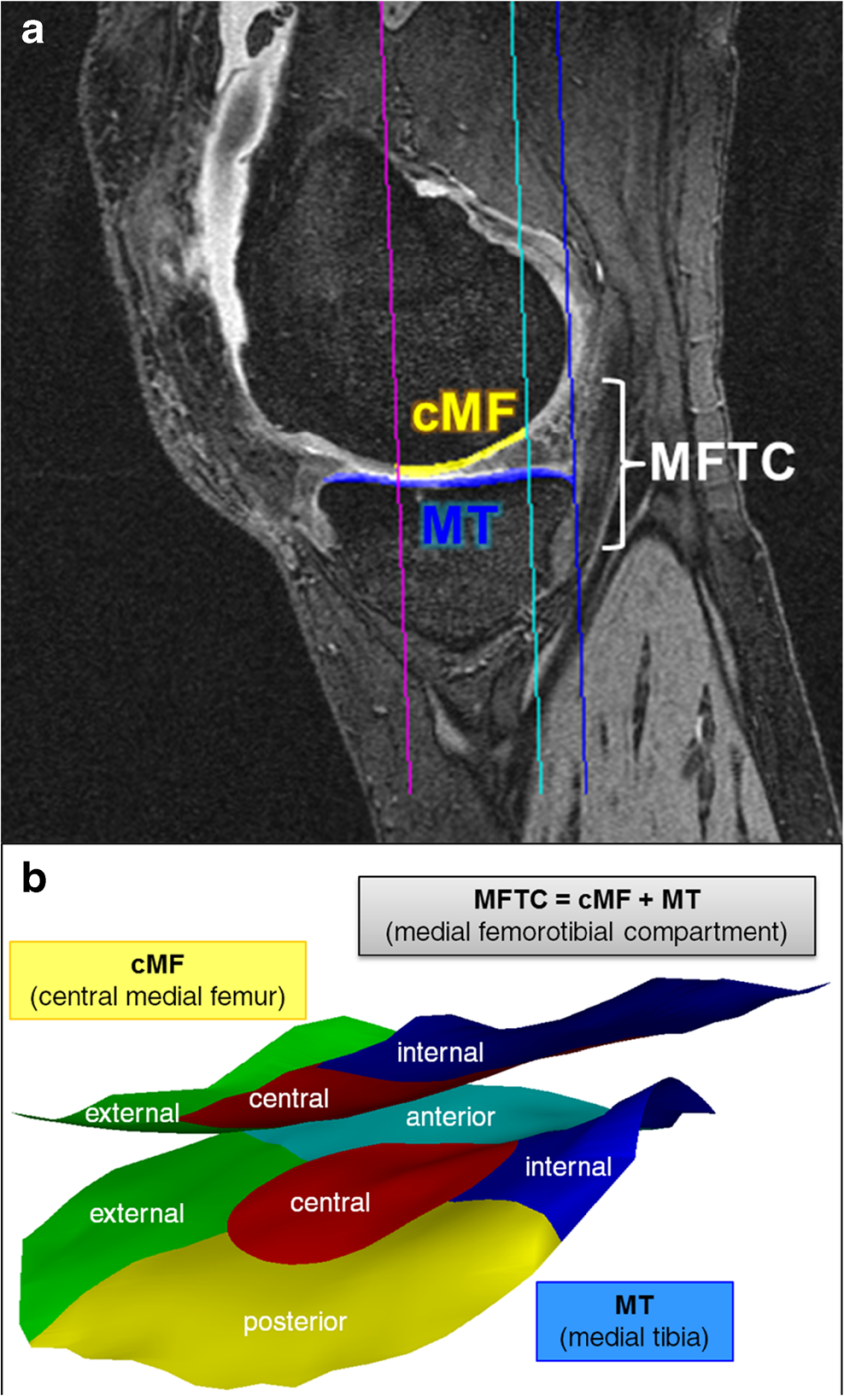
3D analysis of cartilage thickness. MR imaging based, quantitative 3D analysis of the cartilage in the medial femorotibial compartment (MFTC): a sagittal MR image from a double echo steady state sequence (with water excitation) with MFTC cartilage segmentation (cMF = central medial femur; MT = medial tibia); b 3D reconstruction of the medial tibial and weight-bearing femoral cartilage including subregions
